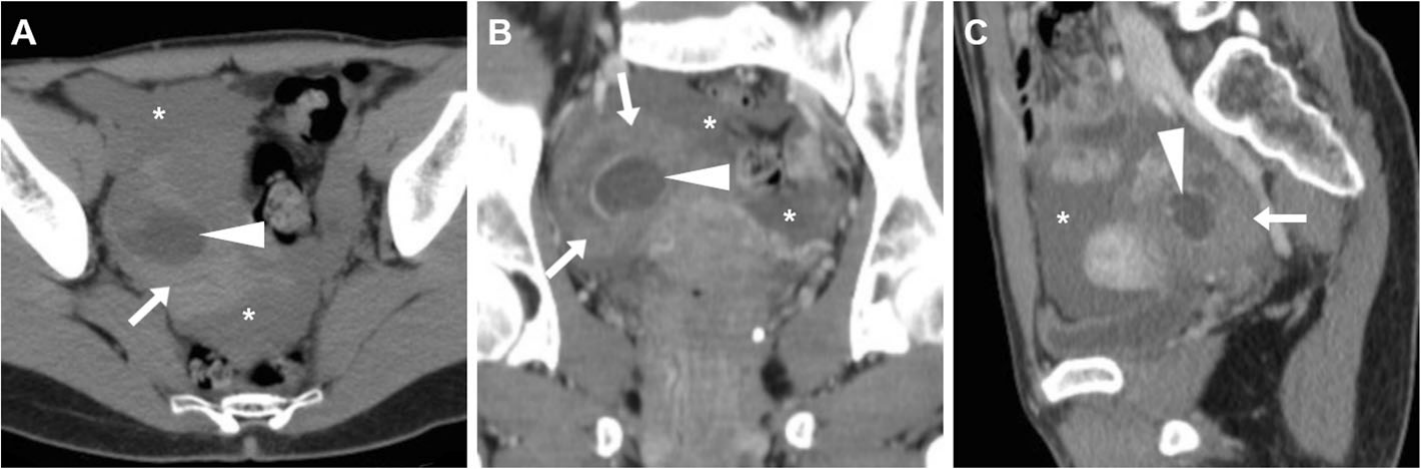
Haemoperitoneum shown at CT (a–c) as pelvic effusion (asterisk) with attenuation higher than that of water, caused by ruptured corpus luteum seen as a 3-cm right adnexal cystic lesion (arrowheads) with enhancing wall, surrounded by high-attenuation ‘sentinel clot’ (arrows)
